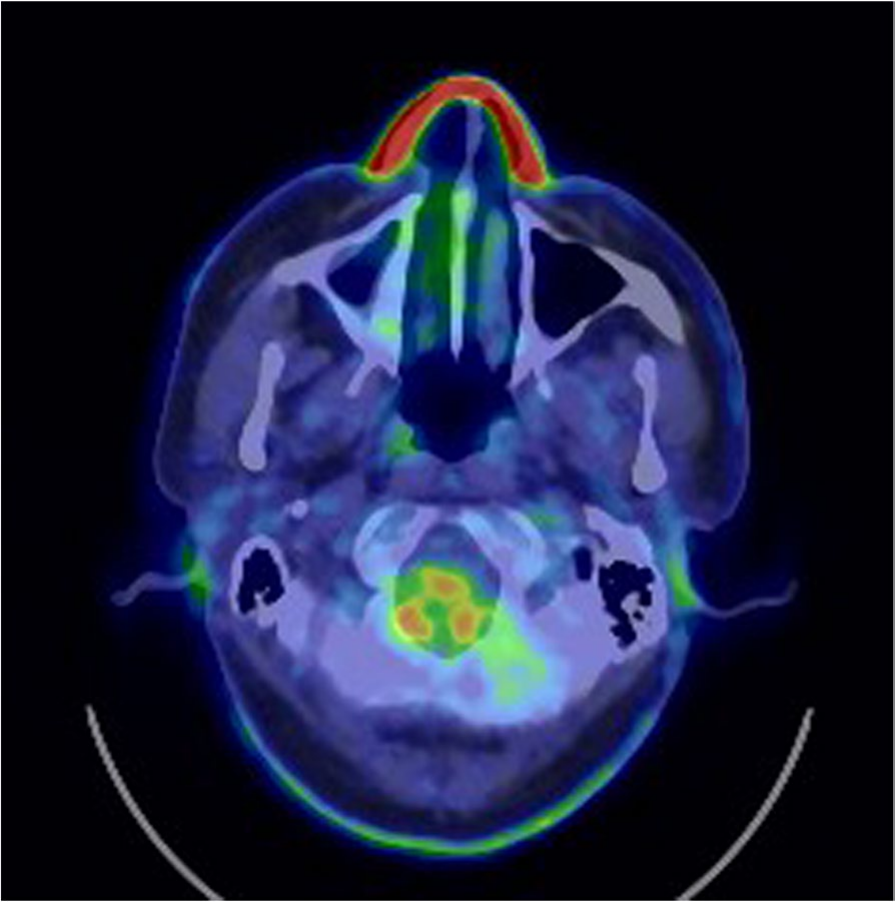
PET-CT taken 3 weeks before admission showed FDG accumulation in the nasal root
